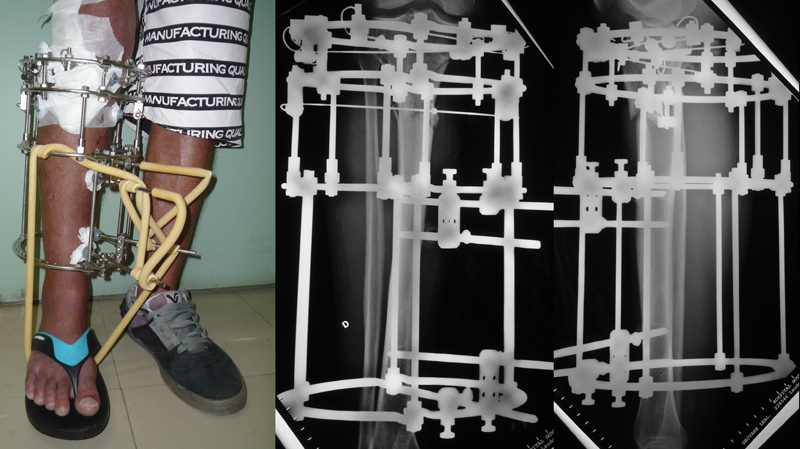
Clinical and radiographic images demonstrating the simplified assembly in a patient included in the study.
